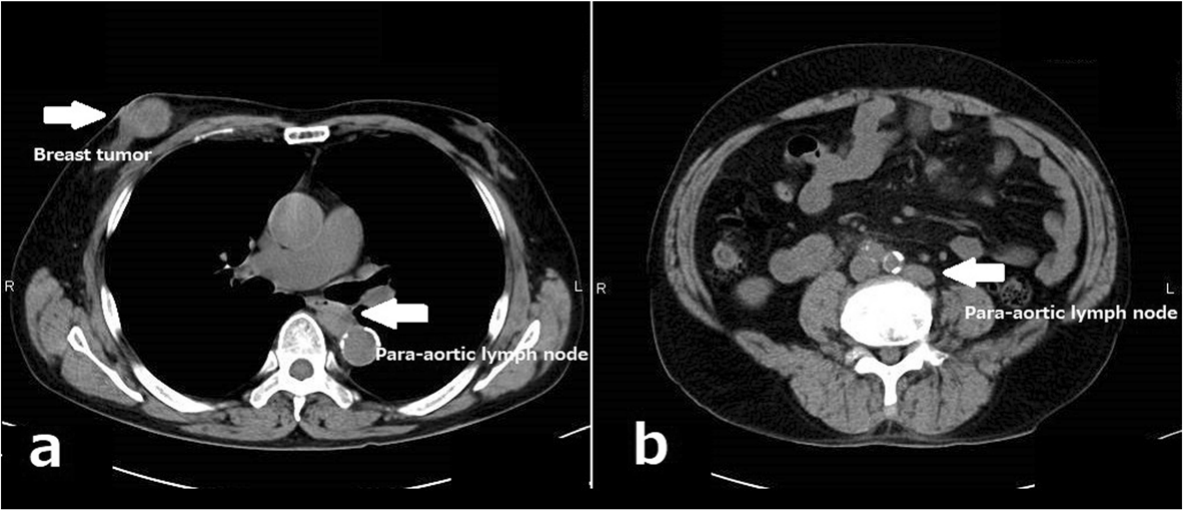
Chest and abdominal computed tomography. Legend: Computed tomography showed the right breast tumor (a) and the para-aortic lymph nodes enlargement which had not changed for several months (a, b)
